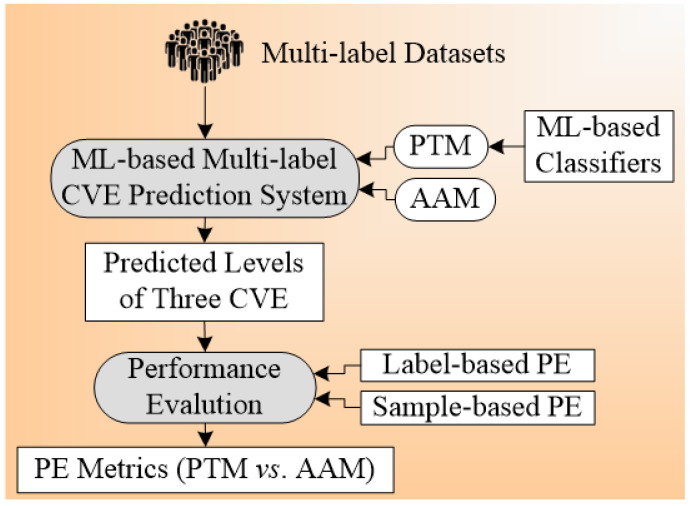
Architecture for multi-label-based CVD risk classification using carotid ultrasound.
